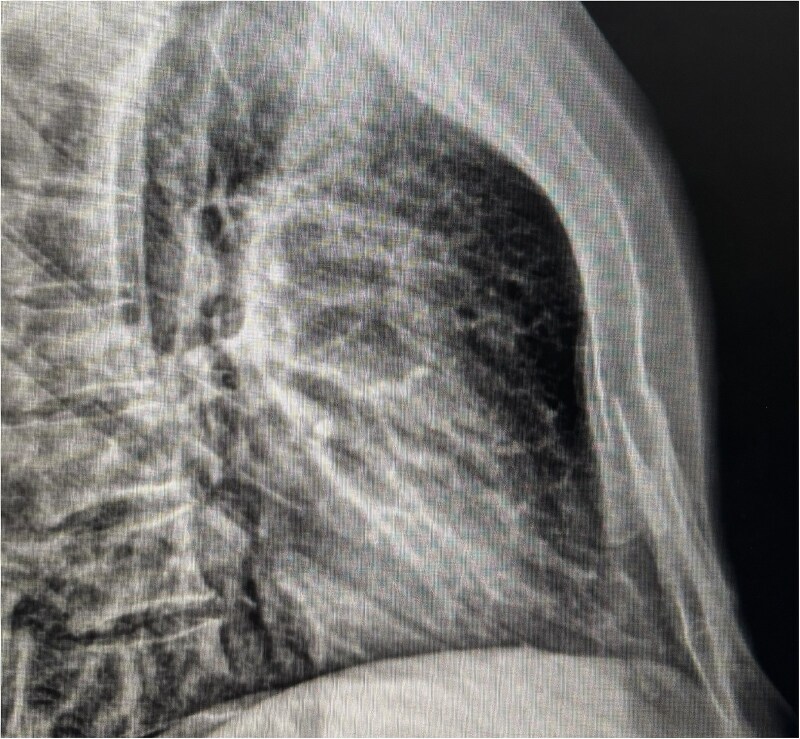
Lateral chest X-ray showing cortical discontinuity in the midportion of the sternal body (red arrow), indicative of an oblique fracture.
